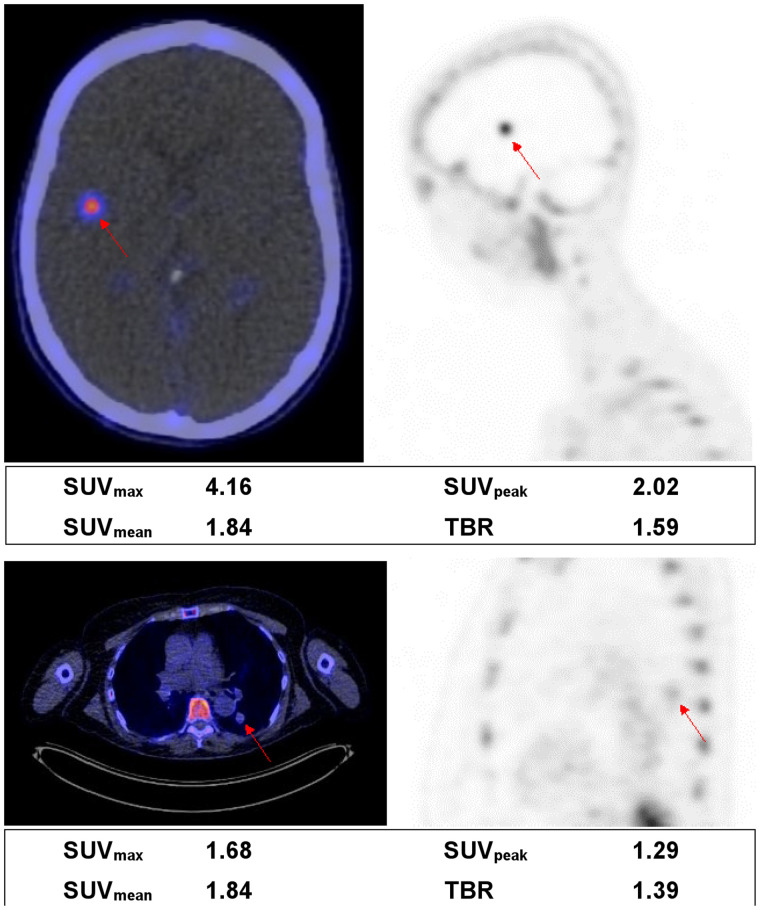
Examples of a brain lesion (top) and a lung lesion (bottom). Fused PET/CT transverse images (left) and PET sagittal images (right) are shown. Semiquantitative measurements are reported underneath images. TBR is calculated as lesion SUV_peak_ divided by SUV_mean_ in aortic blood pool.
